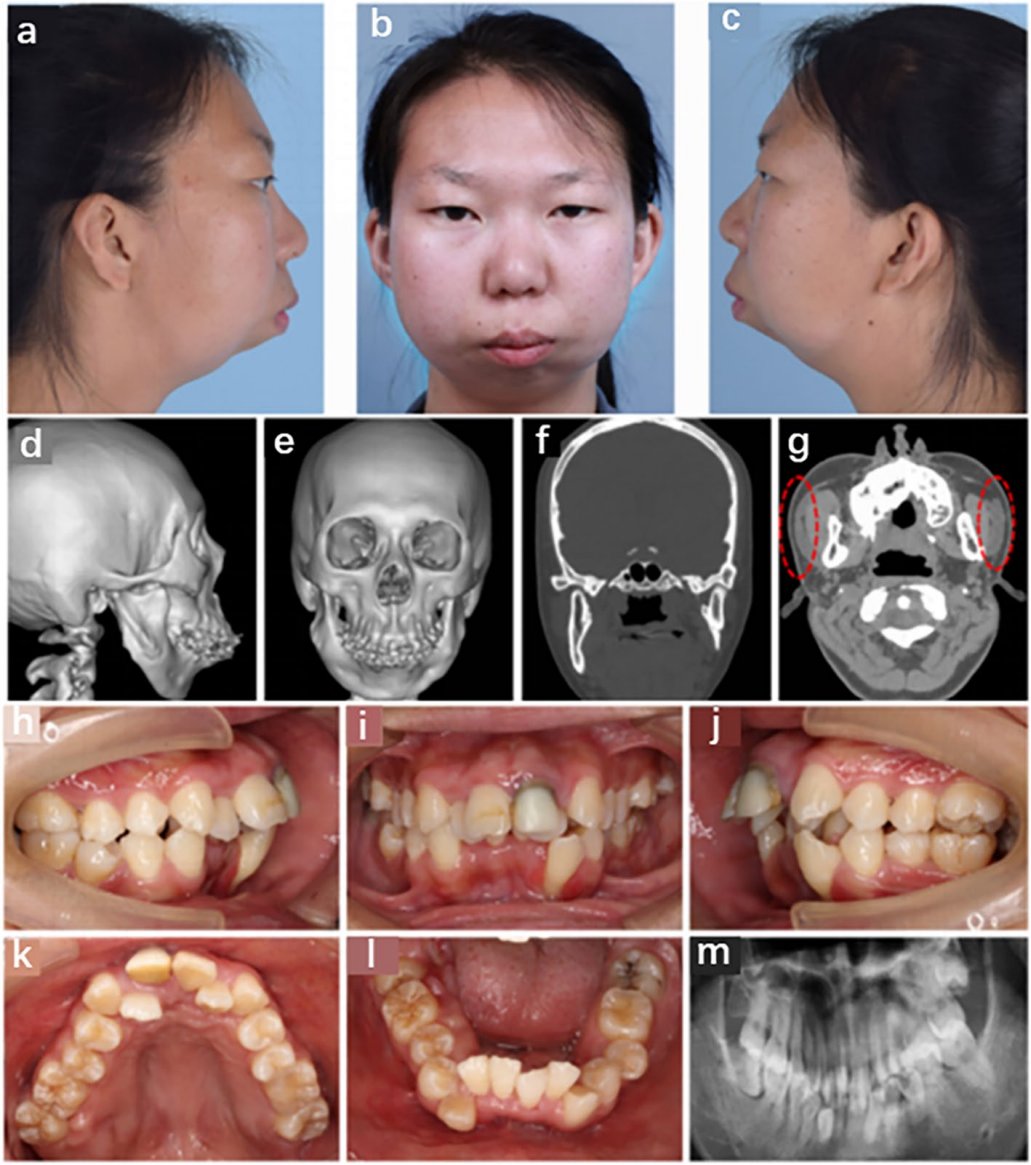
Phenotypic presentation of the patient. a-c The side view of the face showing micrognathia, full cheeks, and round face shape. d-f Three-dimensional (3D) reconstruction of computed tomography (CT) data indicating micrognathia, bony prominence of the mandibular rami, and mandibular condyle hypoplasia. g The red dashed lines indicate a heterotopic parotid gland. h–l Patient’s intraoral view showing deep the overbite and overjet of anterior teeth, disordered tooth, and crowded dental arch. m The panoramic radiograph shows severe malocclusion
